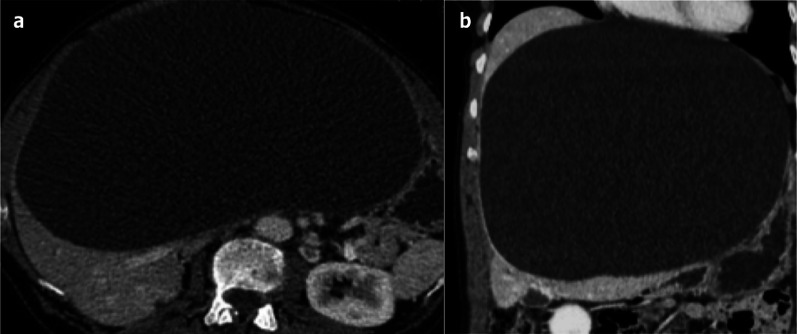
Very large simple hepatic cyst in a symptomatic 64-year-old female with abdominal pain. a Axial and (b) coronal computed tomography on portal venous phase shows a very large simple hepatic cyst. The cyst had grown progressively for a certain number of years and was eventually treated by laparoscopic fenestration, resulting in pain relief
